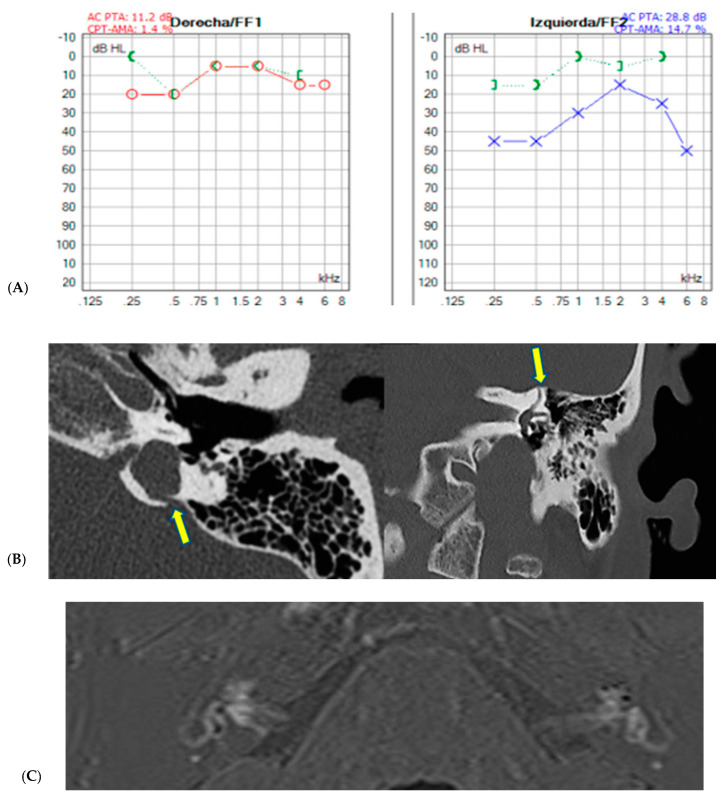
This is the case of a 46-year-old male who was diagnosed at another center with left-sided Ménière’s disease and had shown a poor response to intratympanic dexamethasone treatments. However, due to a clear Hennebert’s phenomenon and the presence of a type B tympanogram with preserved stapedial reflexes in the context of conductive hearing loss, as shown in (A). Both bone conduction showed in green and red and blue representing air conduction. A temporal bone CT scan was performed (B), revealing a double dehiscence at the level of the vestibular aqueduct, involving the jugular vein and the superior semicircular canal (yellow arrows). Additionally, as observed in (C) on the real IR sequence, a hypointensity signal was found in the cochlea, compatible with endolymphatic dilatation at that level (signs of moderate cochlear hydrops in the left ear).
